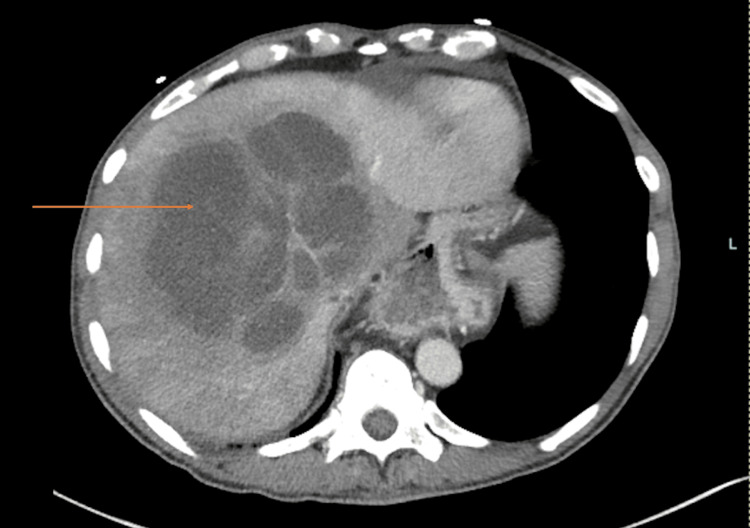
Axial CT scan of the patient's chest, abdomen, and pelvis taken on admission, showing a liver abscess measuring approximately 13 by 12 by 12 cm (indicated by the arrow).
